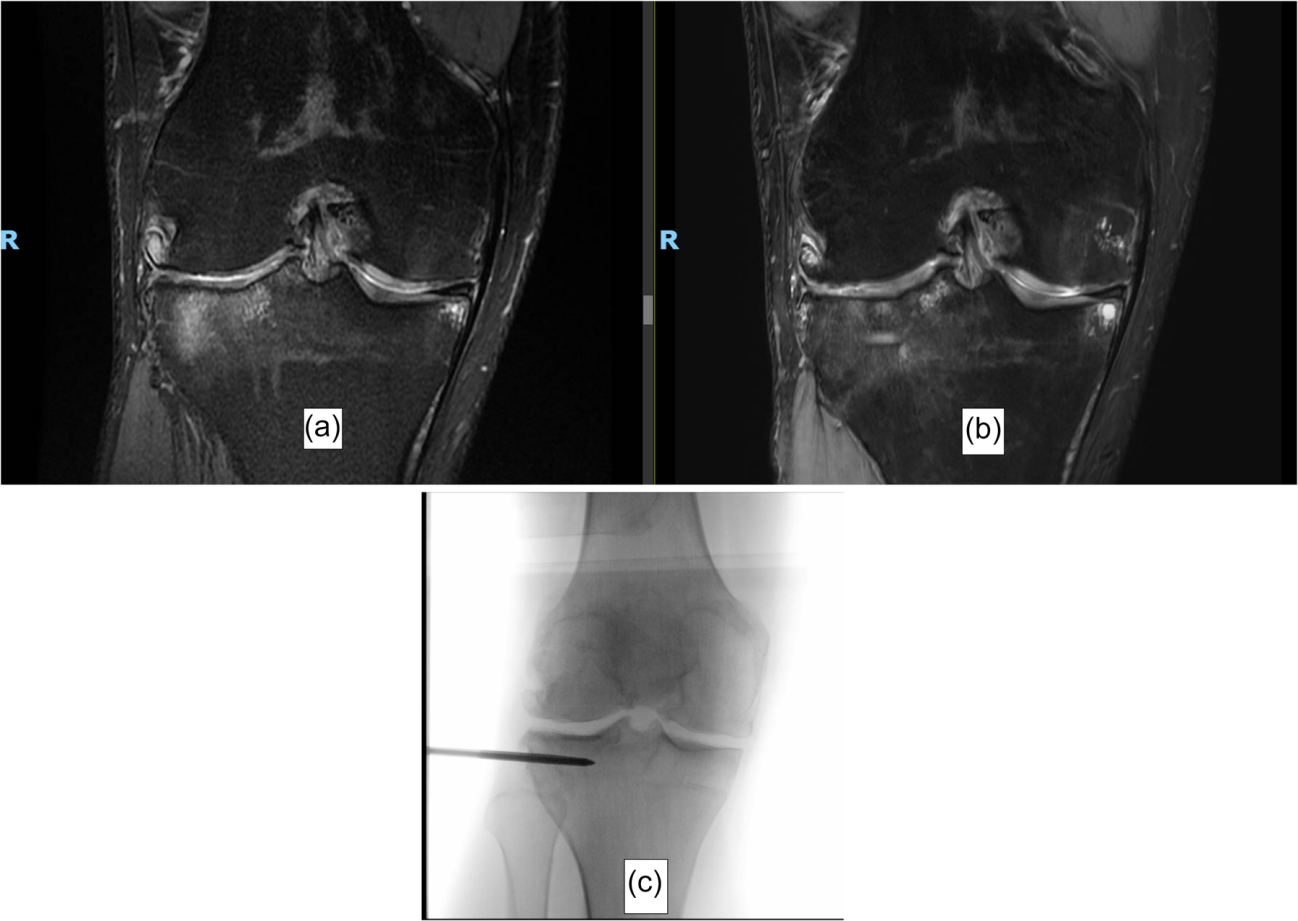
MRI coronal views. (a) Pretreatment, (b) 12 months postintraosseous MSCs injection, and (c) intraoperative procedure image in a 34 years old professional handball player. MRI, magnetic resonance imaging; MSCs, mesenchymal stromal cell.
